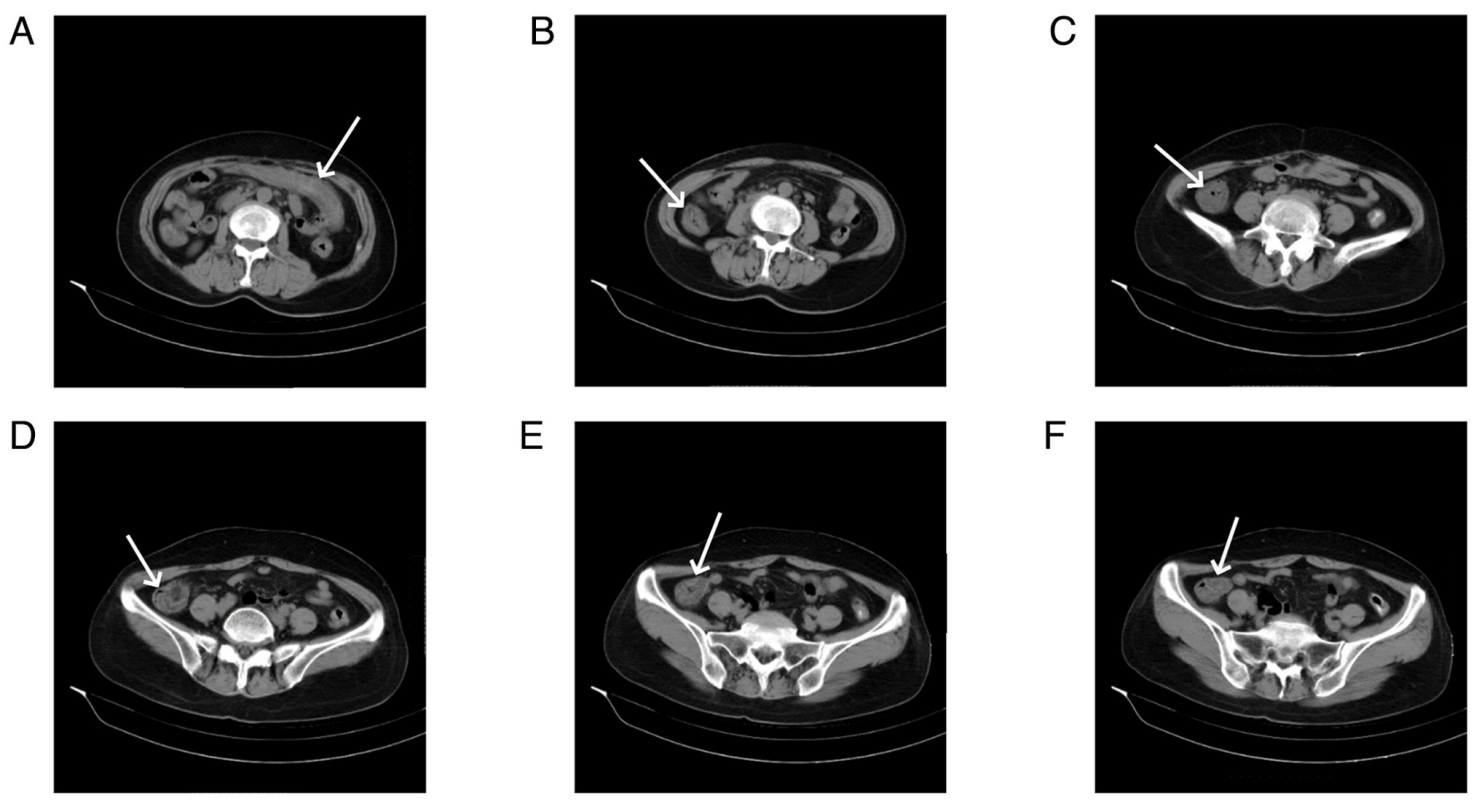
Abdominal computed tomography scan images of a patient with involvement of the right colon. (A) Edema and a thickened bowel wall (arrow) in the transverse colon was observed. A thickened bowel wall (arrows) was observed in the (B-D) ascending colon and (E and F) ileocecal region.
